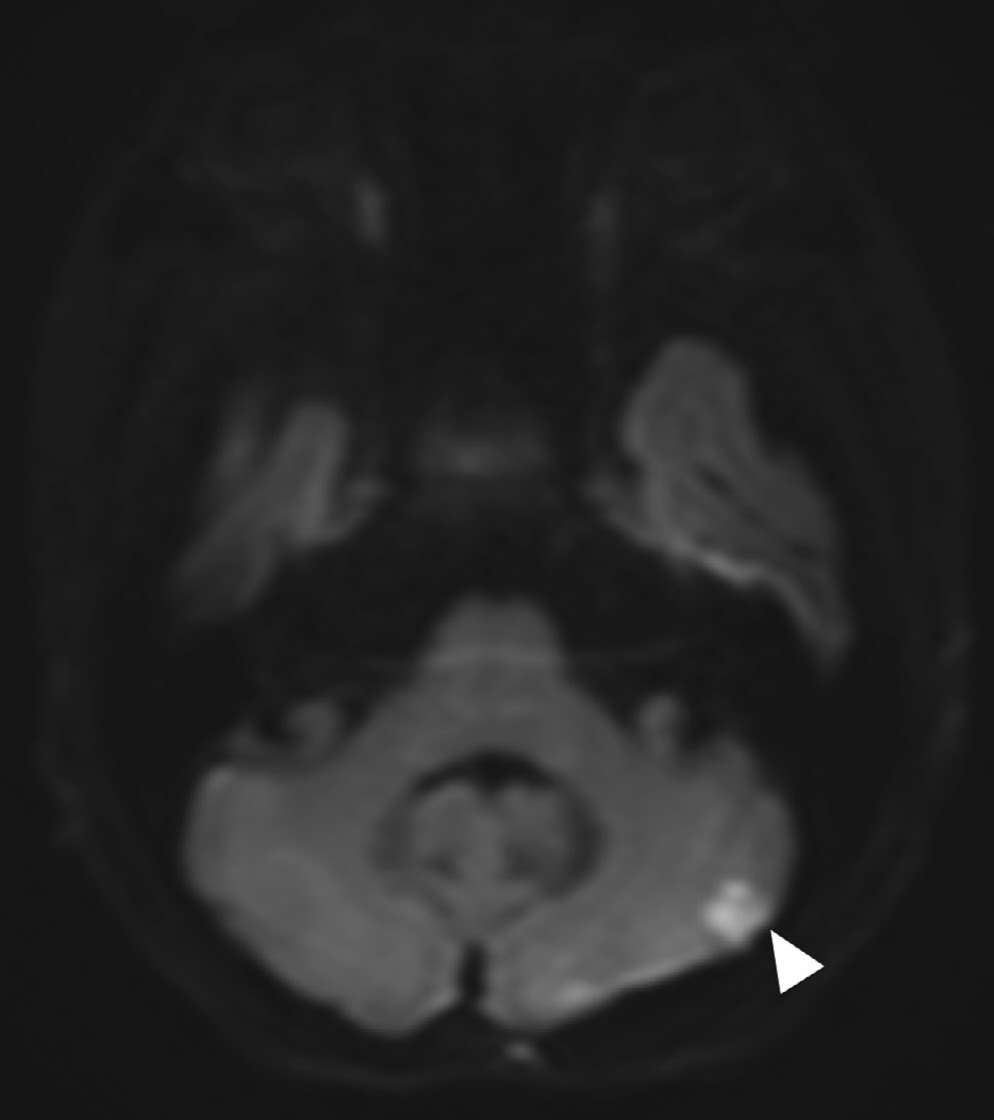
Magnetic resonance imaging of the brain. On diffusion‐weighted images, there is a high‐signal area on the left cerebellum (arrow head)
